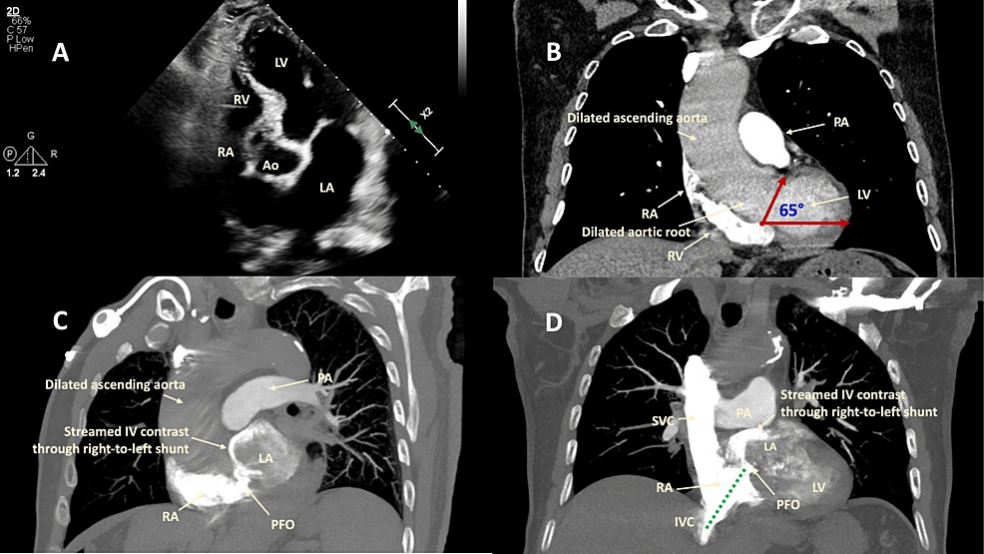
TTE and contrast-enhanced chest CTA with pulmonary embolism protocol. (A) TTE apical five-chamber view with dilated aortic root and ascending aorta covering the RA. The RV appears of normal size without any structural signs of pulmonary hypertension. The heart has an offset axis with counterclockwise rotation secondary to the dilated aortic root and the ascending aorta. Chest CTA image with pulmonary embolism protocol in coronal (B and D) and oblique (C) views showing the horizontal alignment of the aortic root and fusiform ascending aortic aneurysm (increased aortic angulation of 65°), reorienting the PFO with the inflow from the IVC (green dotted line). Streaming of intravenous contrast from the RA into the LA is visualized due to the flow-directed, right-to-left interatrial shunt despite normal right heart chambers (C and D). TTE, transthoracic echocardiogram; CTA, computed tomographic angiography; PFO, patent foramen ovale; RA, right atrium; LA, left atrium; Ao, aortic root; RV, right ventricle; LV, left ventricle; PA, pulmonary artery; SVC, superior vena cava; IV, intravenous
